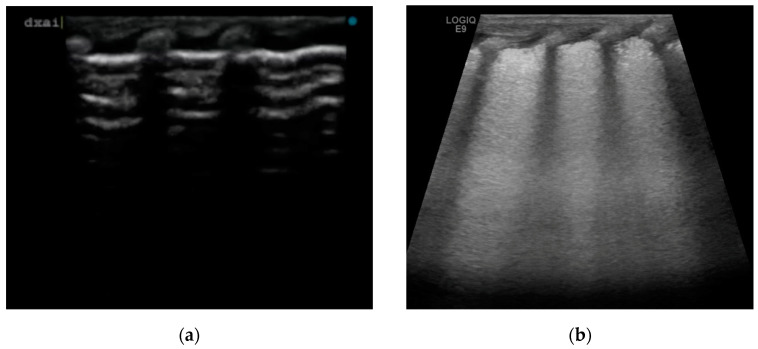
Lung ultrasound images: (a) normal lung, with A-line pattern; (b) diffuse coalescing B-line pattern (white lung), with pleural thickening with some interruption spots and the disappearance of A-line.
